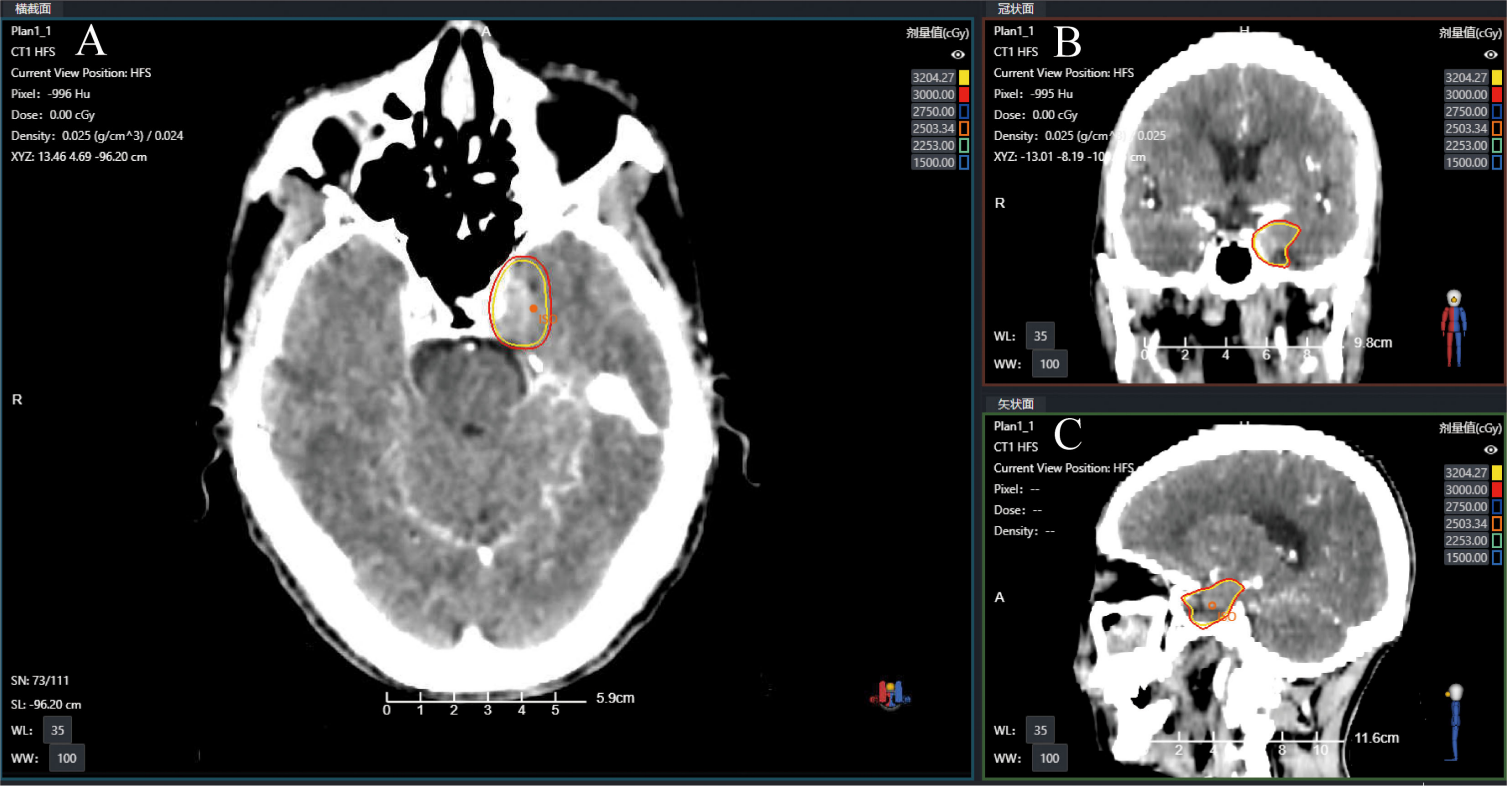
Radiotherapy treatment planning CT images showing the target with isodose lines in (A) axial (B) coronal, and (C) sagittal views.
